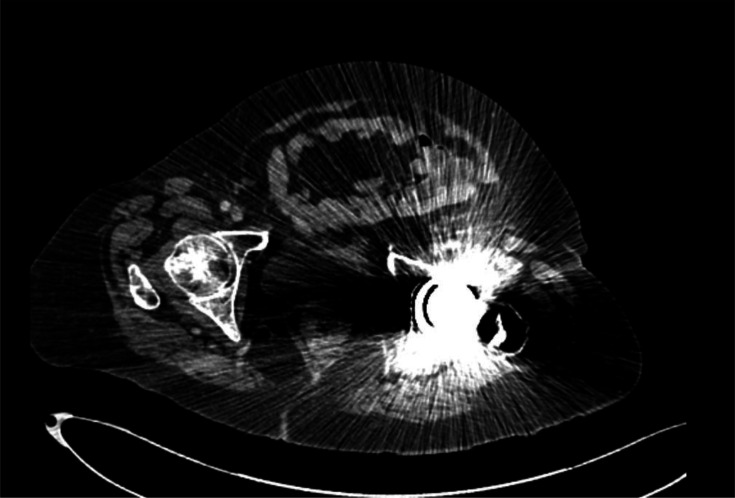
CT abdomen/pelvis showing evidence of colitis in the sigmoid colon and inflammatory changes.
